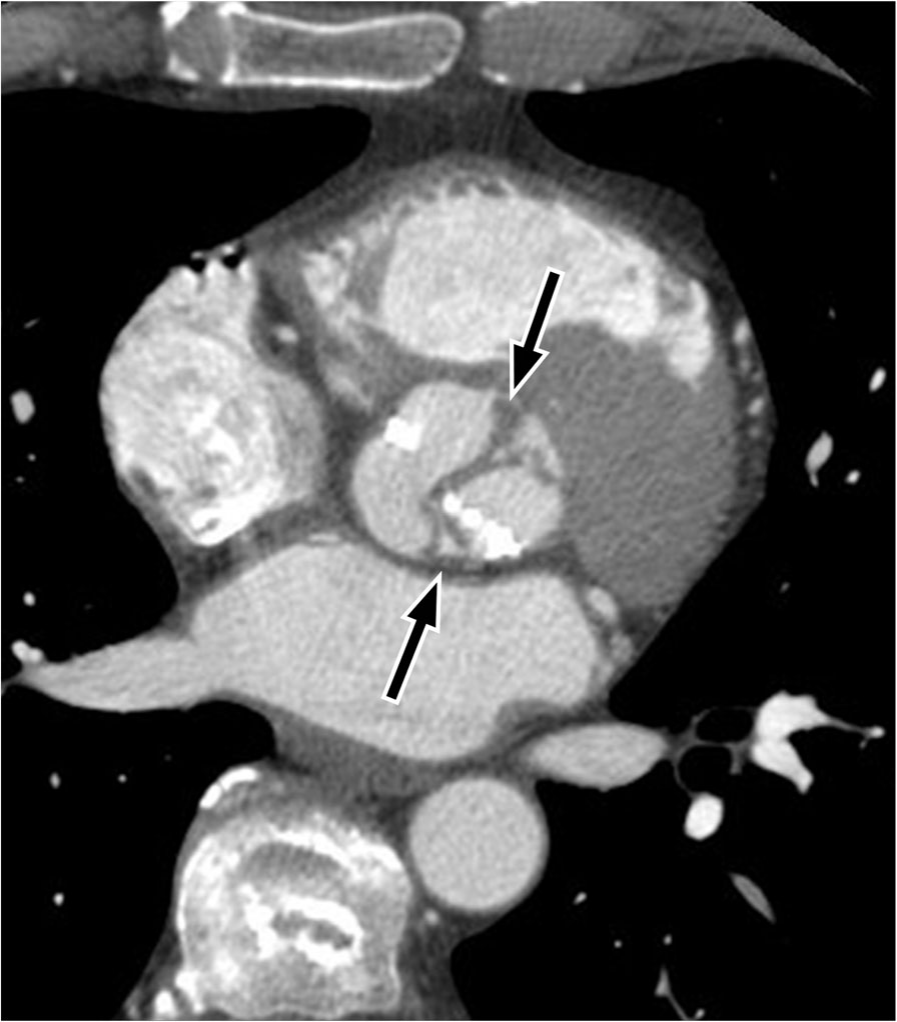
Axial contrast CT shows a bicuspid aortic valve (black arrows) in a 62-year old man being worked up for a widened mediastinum. The valve leaflets are thickened and calcified
